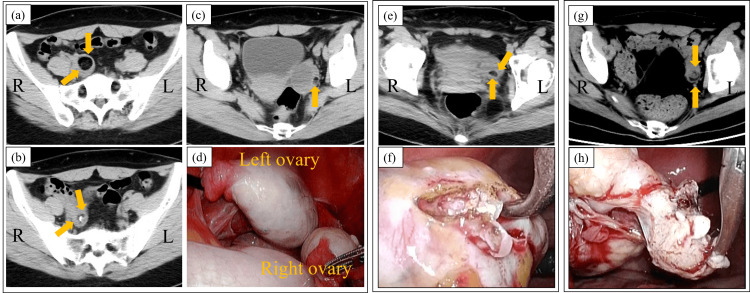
CT and surgical findings during the clinical course. (a-c) Pelvic CT performed at admission revealed bilateral ovarian cysts with fatty components (yellow arrows): two in the right ovary and one in the left, suggesting the presence of bilateral teratomas. One of the cysts also contains calcification, a common finding in teratomas. (d) On day 3, right salpingo-oophorectomy and left ovarian cystectomy were performed. During the procedure, a single large cyst in the left ovary was clearly identified. However, the cysts intended for removal could not be located in the right ovary. Pathological examination confirmed the presence of mature teratomas in the right ovary, while the left cyst was identified as a luteinized follicle. (e) A pelvic CT performed on day 43 confirmed a cyst with a fatty component (yellow arrows), which was the same in size and location as the CT taken at admission. (f) The second surgery was conducted to remove the residual teratoma. During the operation, the teratoma was recognizable by its yellowish appearance on the ovarian surface. Removal of the whole ovary was avoided to preserve fertility. Pathological examination confirmed the correct resection of the tumor. (g) Pelvic CT on day 191 found an ovarian cyst with a fatty component (yellow arrows). Recurrent teratoma was diagnosed because the previous CT showed no cyst. (h) In the third operation, the teratoma containing hair was successfully removed.
